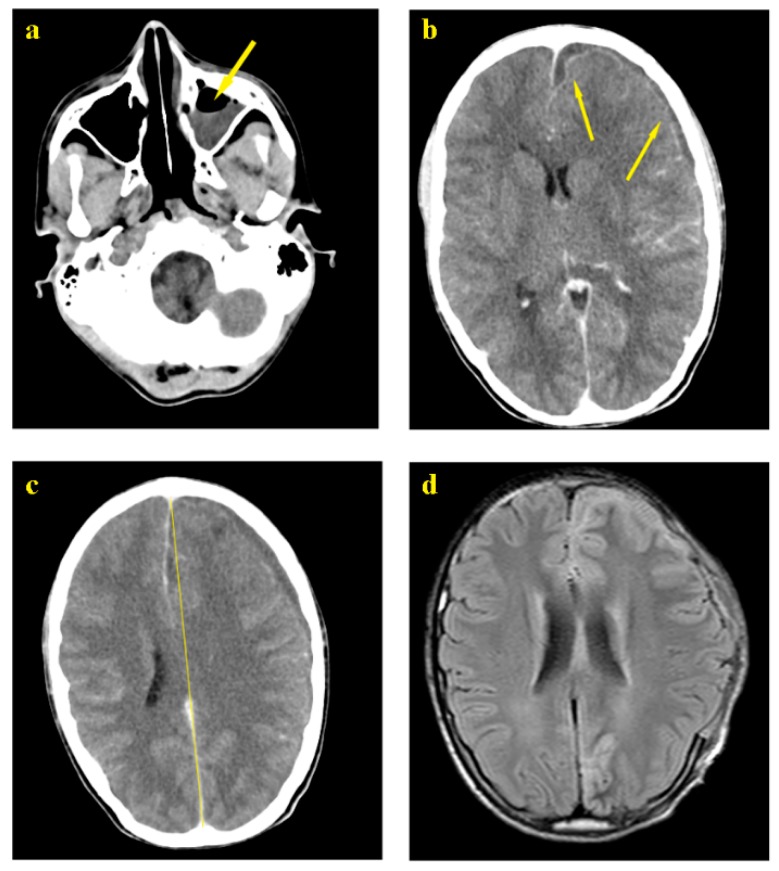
(a) Left ethmoid sinusitis; (b) Meningitis; (c) Meningitis and midline shift front to parietal collection; (d) Brain MRI post decompression (craniotomy).
